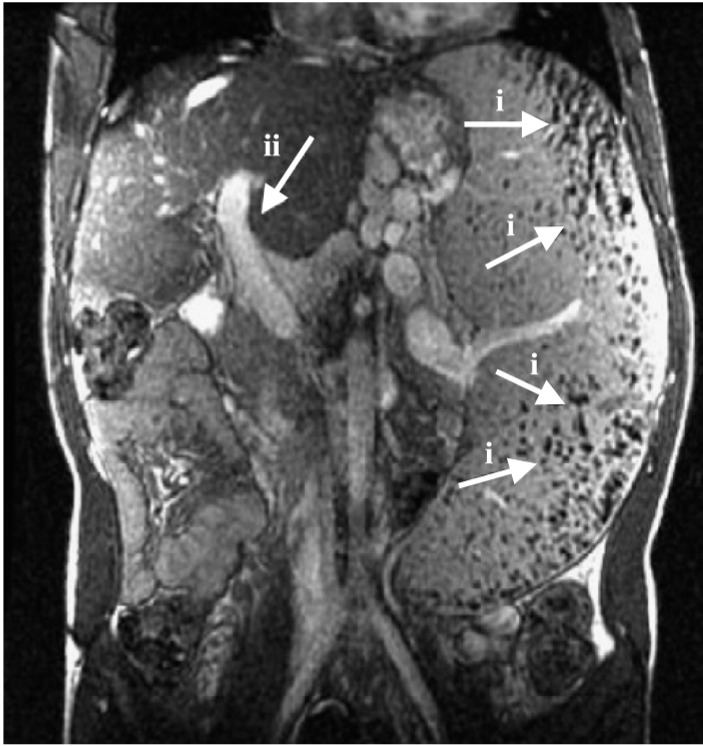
MRI: Gamma-Gandy bodies (siderotic nodules) pointed by arrows labelled “i” in the spleen of a patient diagnosed with hepatosplenicschistosomiasis. Arrow labelled “ii” shows the portal vein. Reproduced with permission [112].
